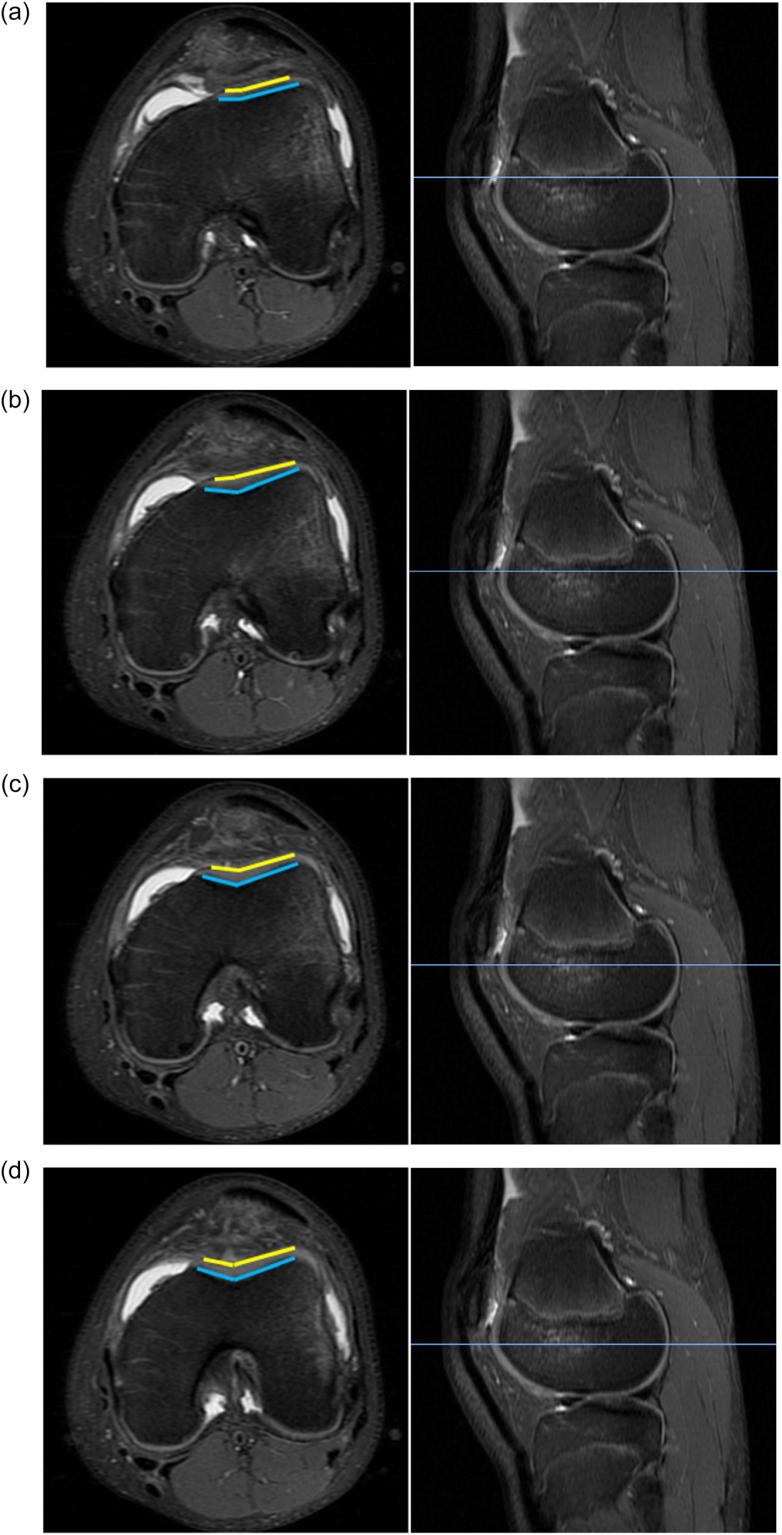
The measurement of (a) proximal SA (SA1), (b) near‐proximal SA, (c) near‐distal SA, (d) distal SA was recorded on T2 weighted MRI. The SA was formed by lines connecting the most prominent portions of the medial and lateral condyles with the deepest point in the TG [10, 15, 29]. The cartilaginous SA was depicted in yellow, and the osseous SA in blue. Created utilising PACS software and Microsoft Word. MRI, magnetic resonance imaging; SA, sulcus angle; TG, trochlear groove.
